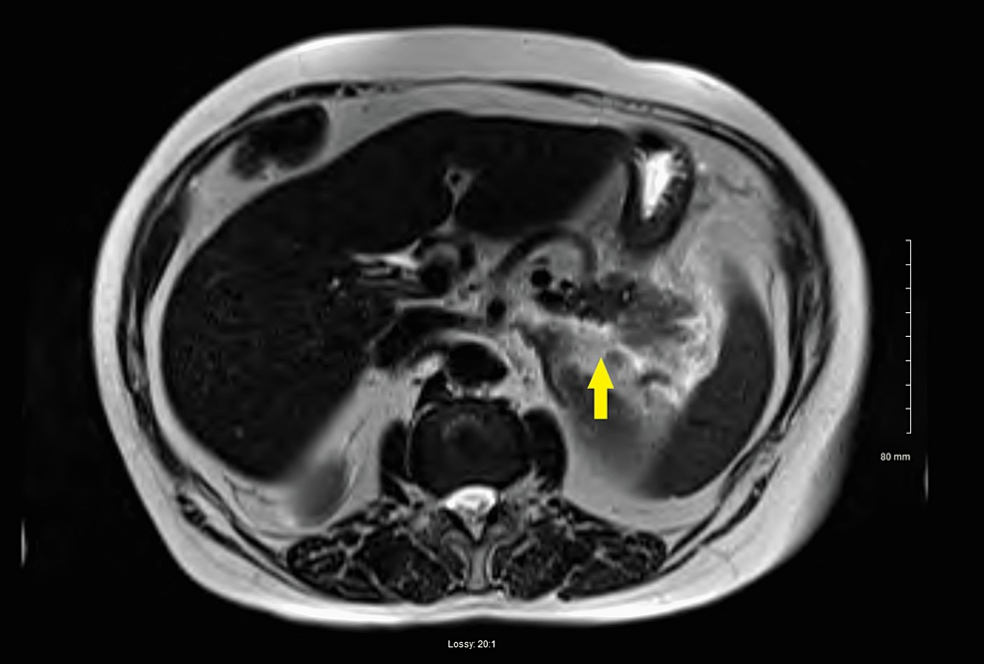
MRI of the abdomen with contrast Arrow is demonstrating pancreatitis.
